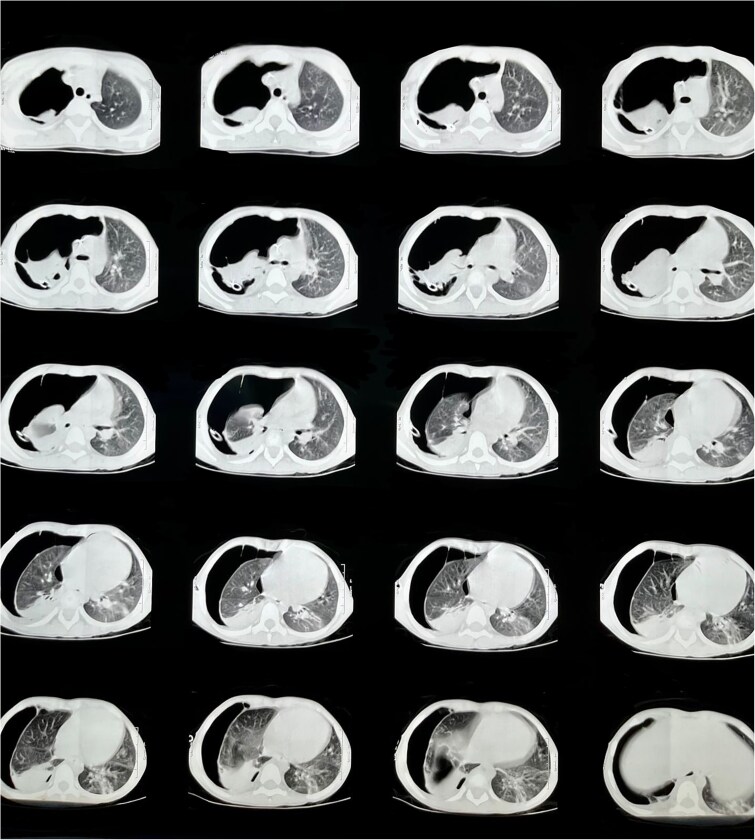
Chest CT scan on lung window showing a hypoplastic right lung.
